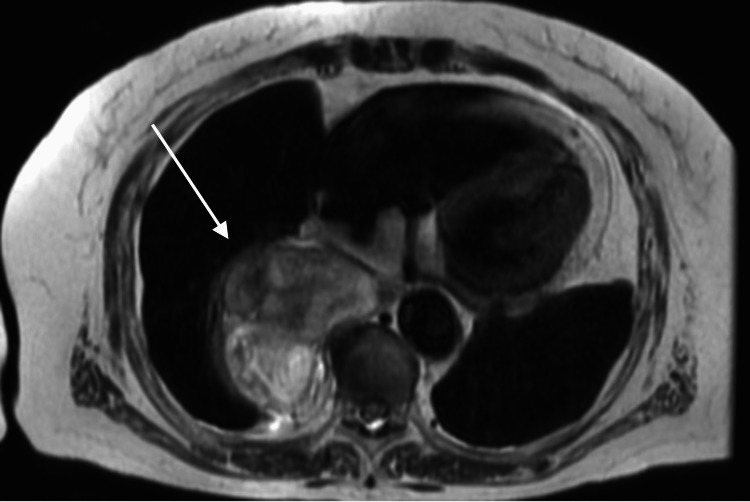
Chest magnetic resonance imaging (T2-weighted imaging) A lobulated mass with a well-defined margin is observed in the right posterior mediastinum, showing uneven mild hyperintensity. The lesion is adjacent to the esophagus, and findings suggestive of a beak sign are observed.
